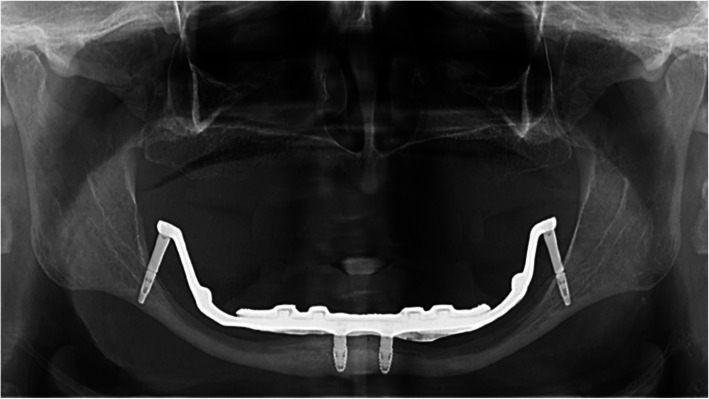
Post denture insertion orthopantomograpy
